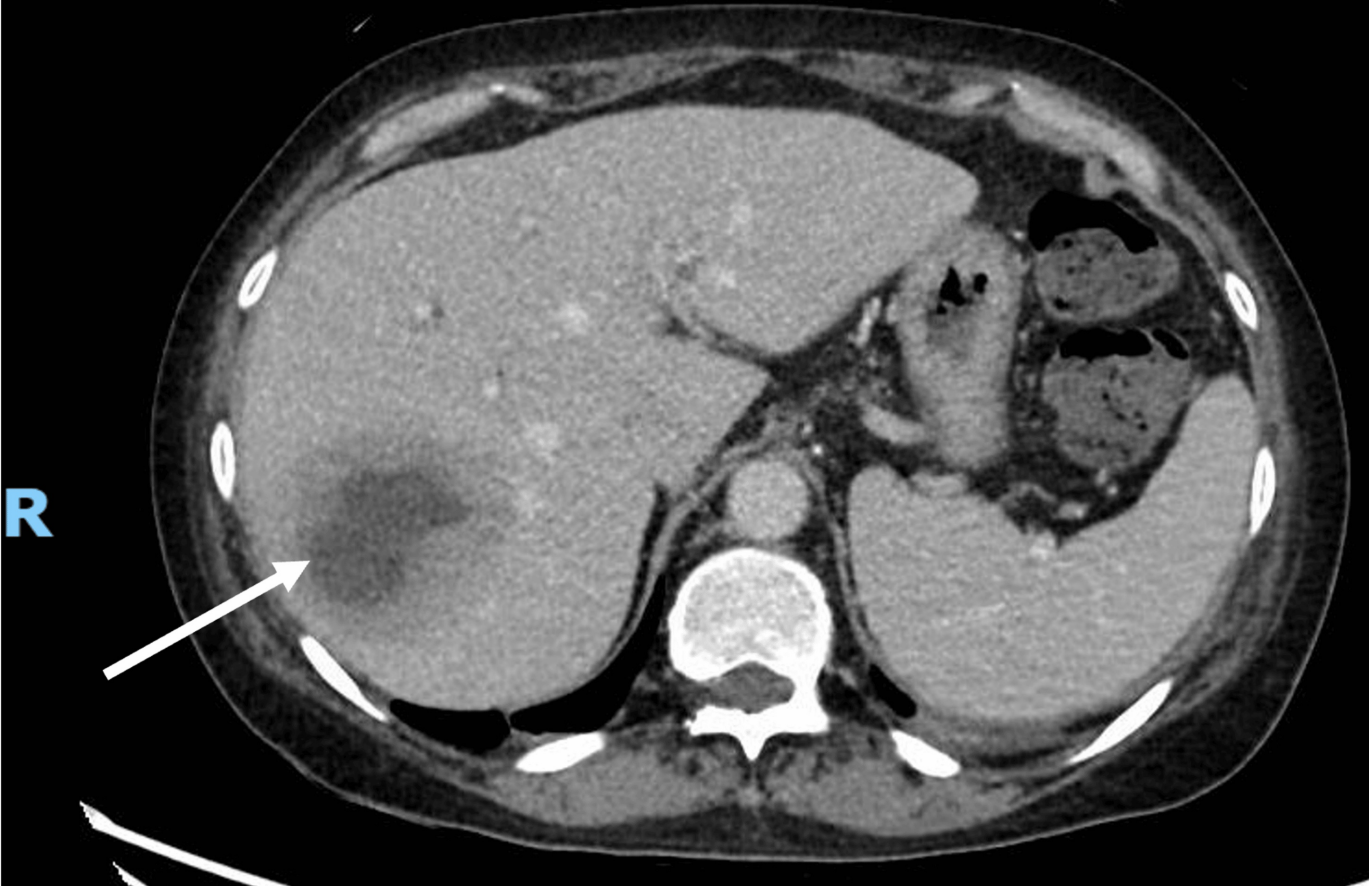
Computed tomography of the chest, abdomen and pelvis demonstrating right hepatic lobe heterogenous lesion with central hypodensity (arrow)
